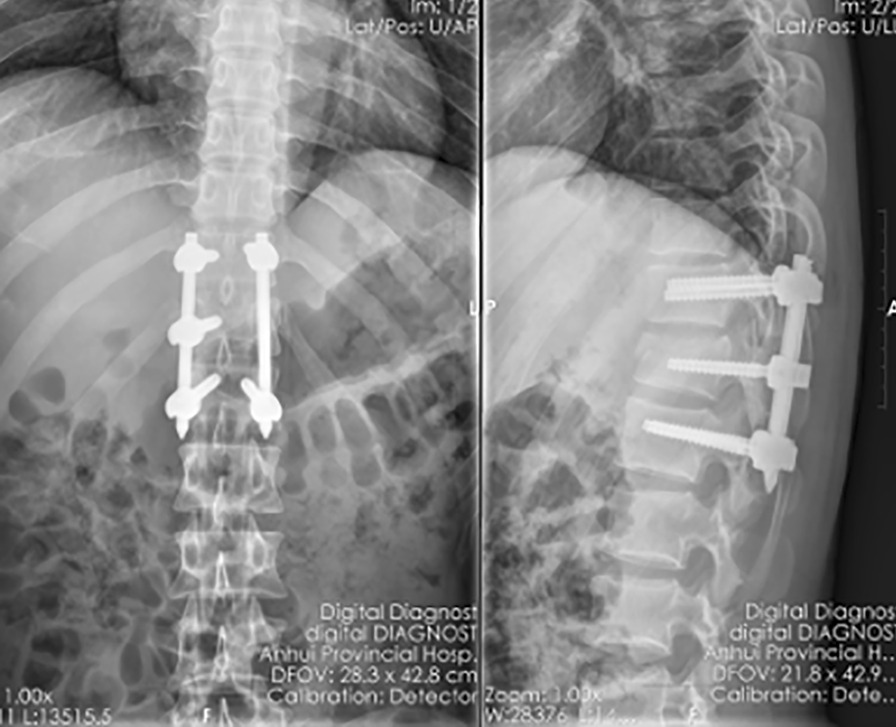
Postoperative radiograph. The fracture was reduced and the position of the screw was good
